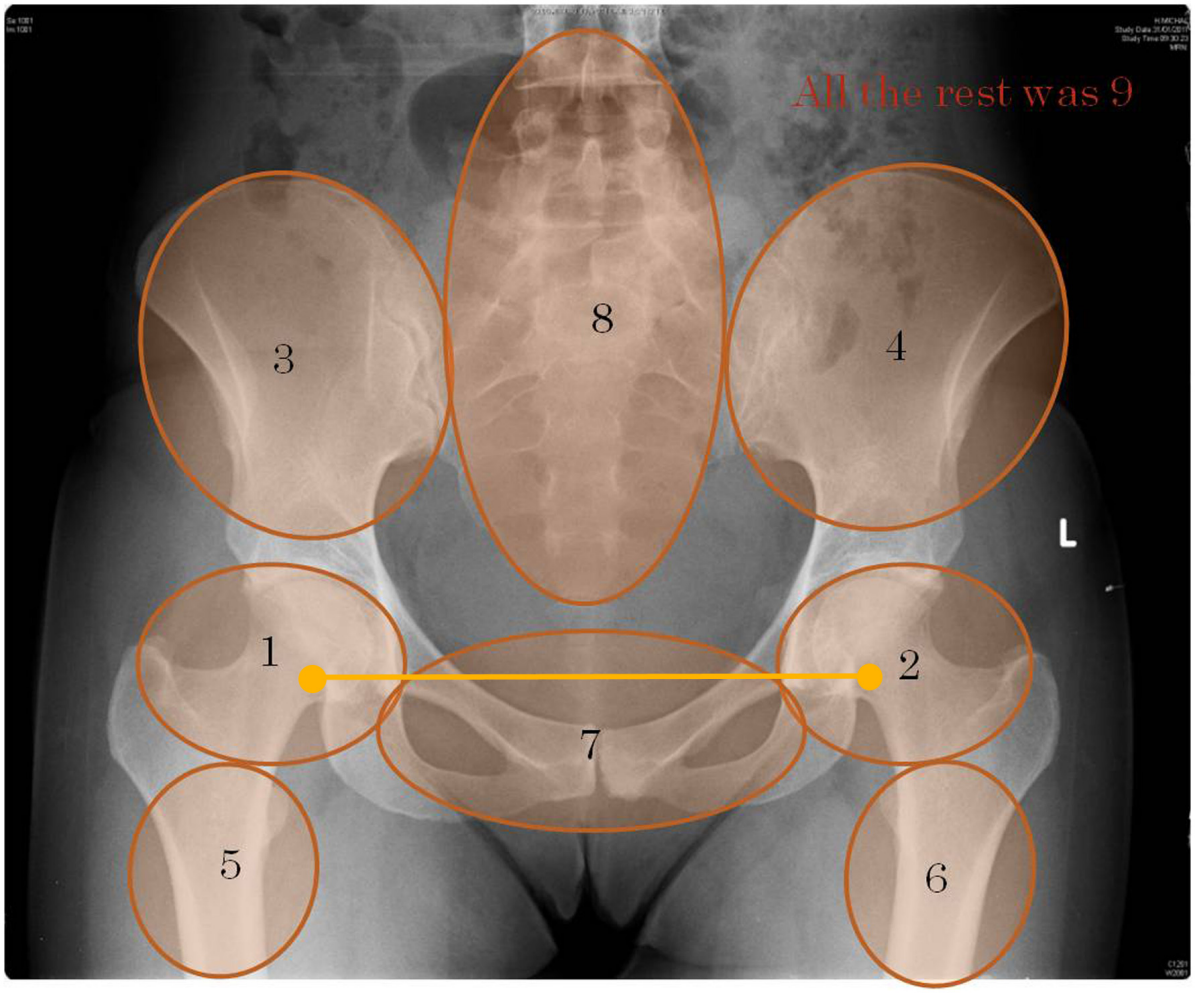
A comparison and RQA areas example. A radiograph with a comparison marked by a yellow line. This comparison represents a saccadic movement between two fixations in opposite symmetric areas. The radiograph also represent eight highlighted FOI areas where the unmarked area is considered as the ninth area. These areas were used for the RQA.
